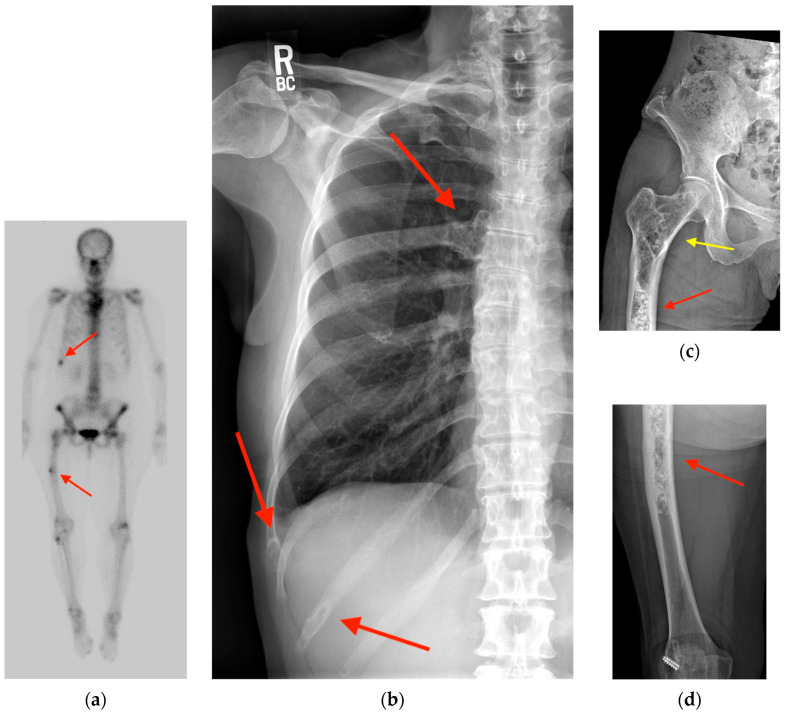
(a) Bone scintigraphy in a 69-year-old woman with urothelial carcinoma shows multiple areas of focal uptake including in the right eleventh rib and the right femur (red arrows). (b) Corresponding chest radiograph reveals multiple “ground glass” expansile lesions in the ribs (red arrows) surrounded by a halo of sclerosis suggestive of polyostotic fibrous dysplasia. Radiographs of the proximal right femur (c) and distal right femur (d) reveal a long intramedullary lesion (red arrows) with a classic Shepherd crook deformity (yellow arrow). This lesion has a denser matrix, correlating with a higher degree of woven bone relative to fibrous elements.
